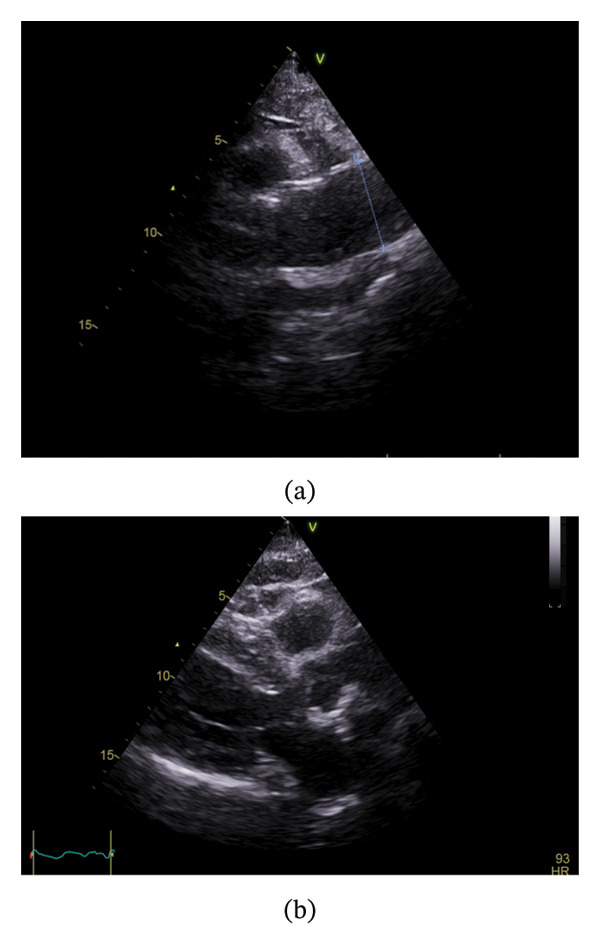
Parasternal long‐axis view in transthoracic echocardiography, (a) at the 3rd intercostal space, showed dilated ascending aorta at the mid‐level as measured 4.1 cm (shown by blue dotted line), in a patient with (b) hypertrophic cardiomyopathy (parasternal long‐axis view at 4th intercostal space).
